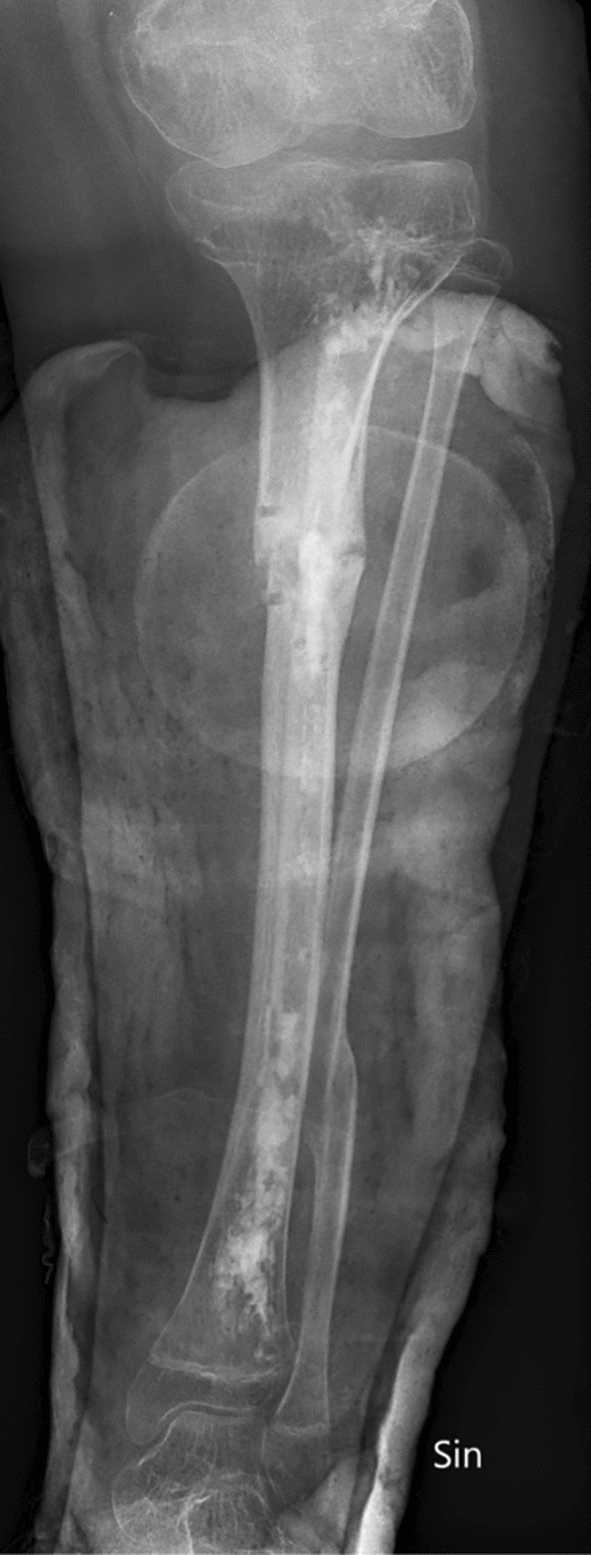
Post-surgery with CERAMEMT^®^ V 2023. X-ray post-surgery in March 2023, with extensive debridement and implantation of CERAMENT^®^ V with vancomycin
